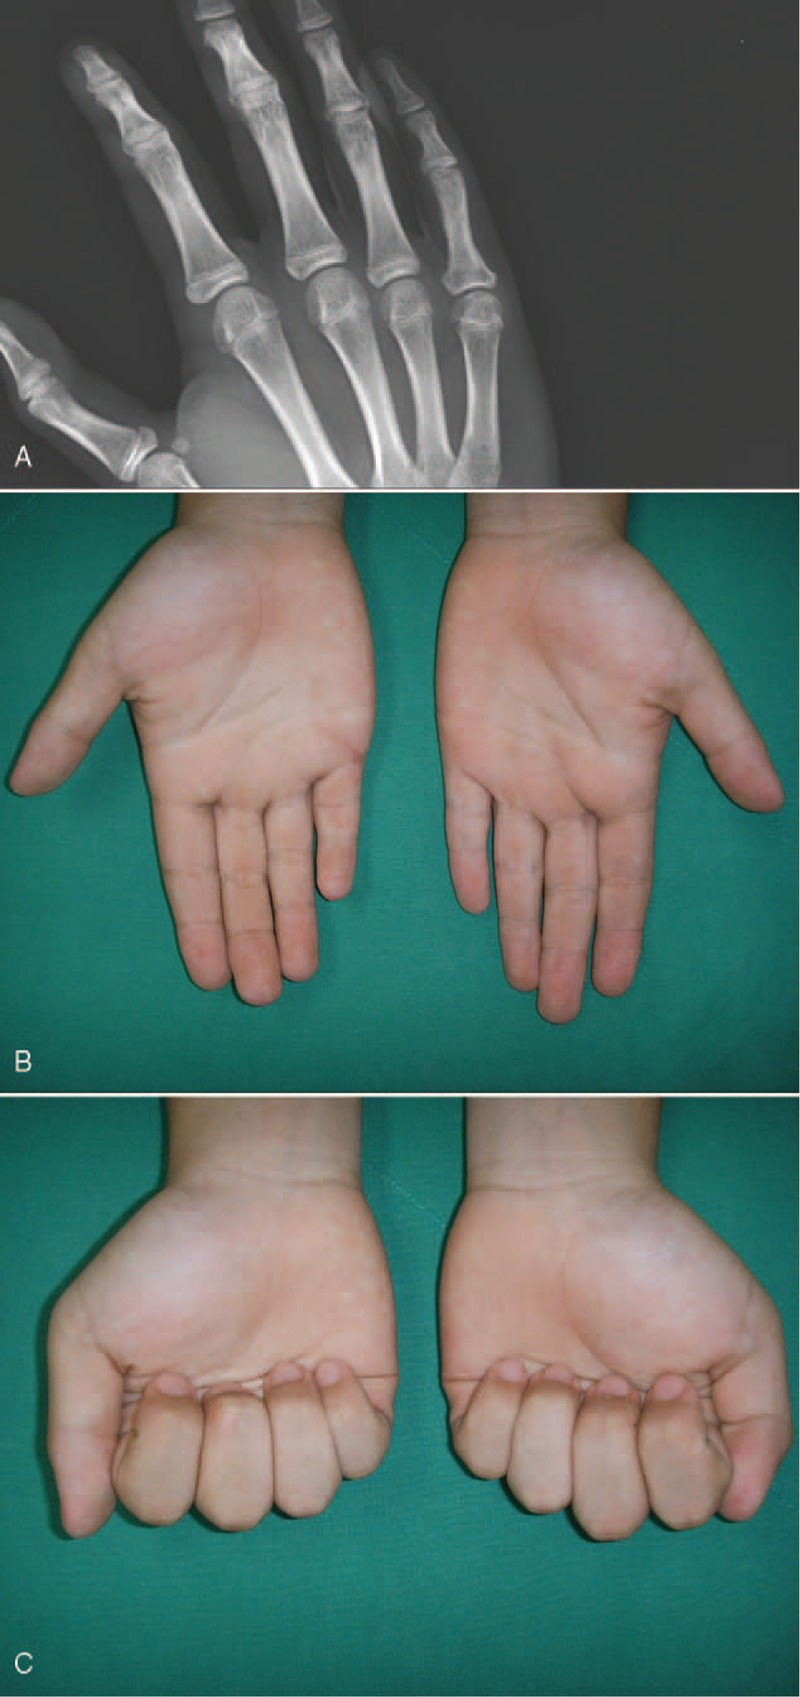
AC (A) An oblique radiograph obtained 12 months after operation shows the growth plate of proximal phalanx of small finger closed naturally. (B, C) Photographs obtained 12 months after operation show a normal range of motion and alignment of a right small finger.
